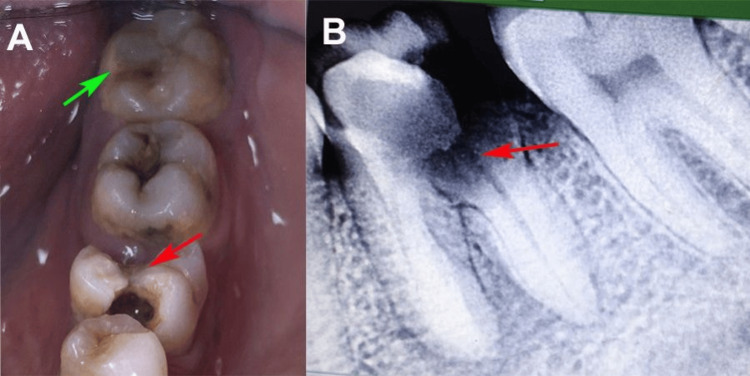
(A) Intraoral image showing grossly decayed left mandibular first molar (red arrow) and intact healthy third molar (green arrow). (B) Intraoral periapical radiograph showing grossly decayed left mandibular first molar (red arrow).
